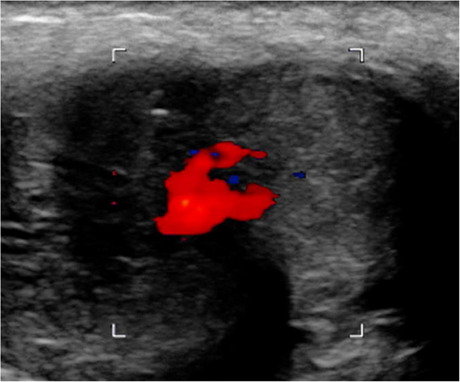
Duplex ultrasound displaying a small correlating pseudoaneurysm in the right cavernous body
